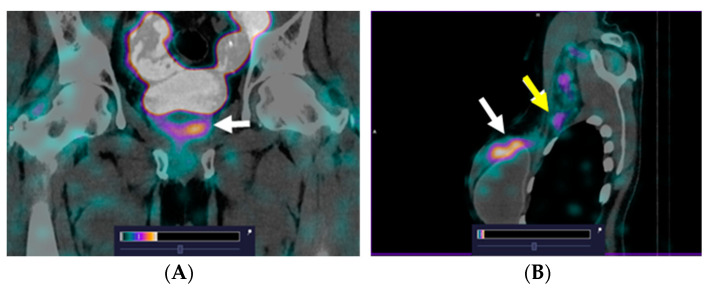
Fused SPECT/CT images of prostate cancer (A) and breast cancer (B) patients 2 h after injection of [^99m^Tc]Tc-maSSS-PEG_2_-RM26. A focus of increased [^99m^Tc]Tc-maSSS-PEG_2_-RM26 uptake is visualized in the prostate gland ((A), white arrow), in the right breast ((B), white arrow), and in the right axillary node ((B), yellow arrow).
